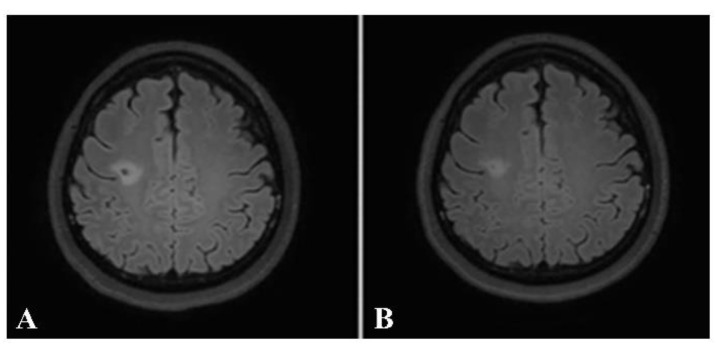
Follow-up axial postcontrast FLAIR images of the same patient after treatment with steroids at 2 and 6 months (A and B, respectively), showing decreased size of the lesion without residual enhancement.
